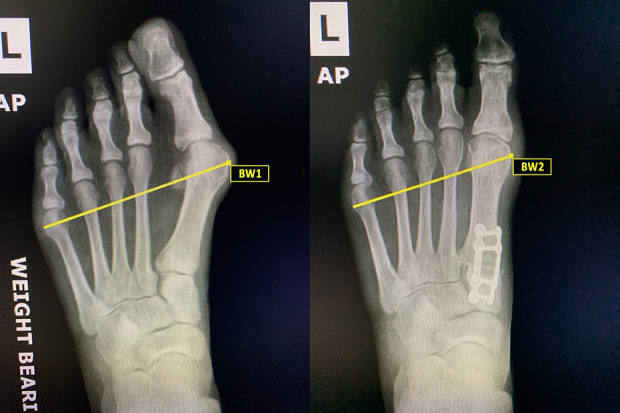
Weightbearing radiographs showing changes in bony width preoperative (BW1) and postoperative (BW2) after triplanar first tarsometatarsal arthrodesis. Bony width was defined as the distance from the most medial aspect of the first metatarsal head to the most lateral aspect of the fifth metatarsal head.
